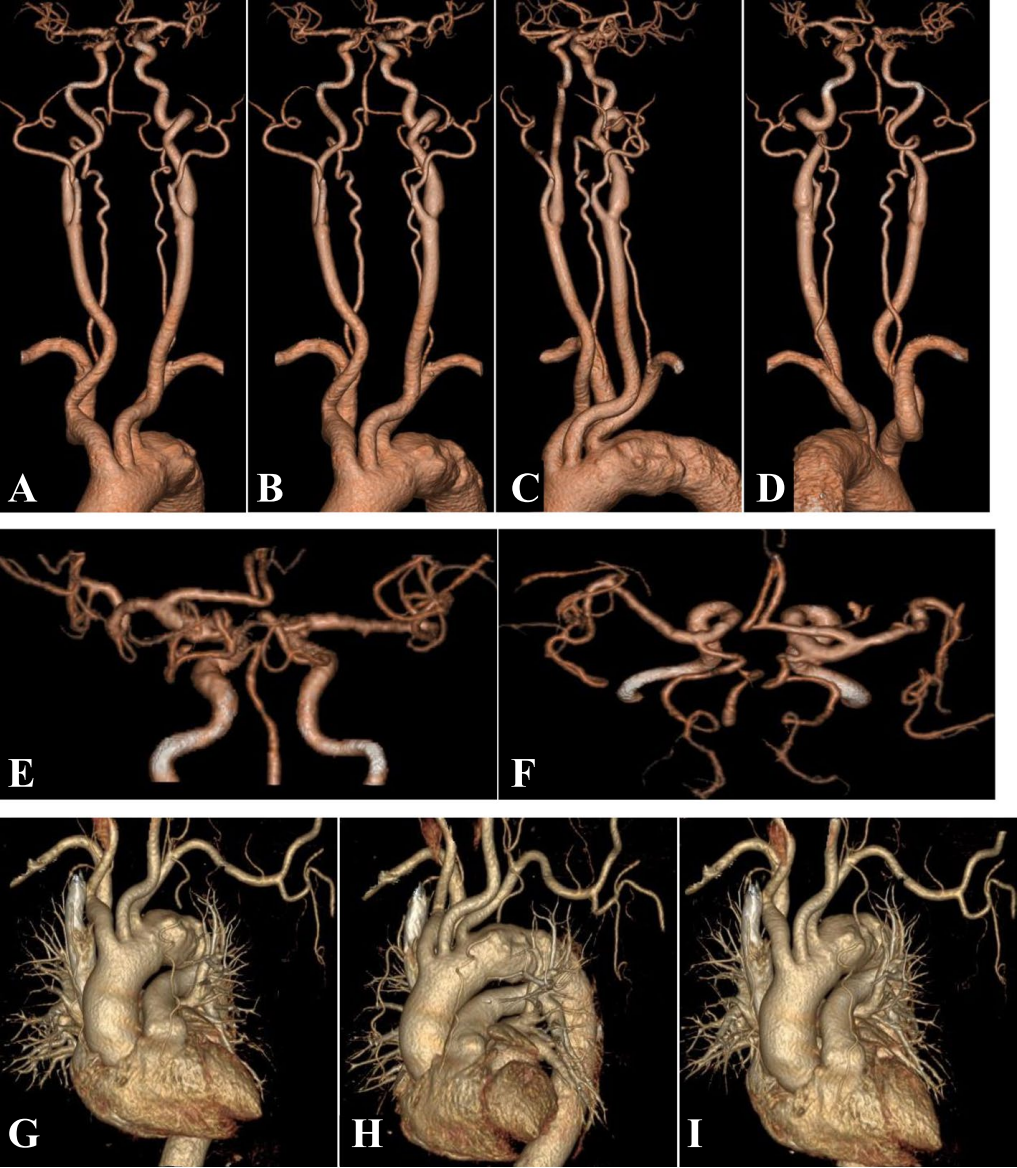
A-I: Head and neck CTA (computed tomography angiography), and chest CTA: noncalcified plaque of the aortic arch with mild stenosis and multiple penetrating ulcers, noncalcified plaque and mild stenosis of the descending aorta; Noncalcified plaque with mild luminal stenosis in the proximal segment of the left common carotid artery and the initial segment of the left internal carotid artery; mixed plaque in the left carotid sinus and mild luminal stenosis; Calcified plaque in the right carotid sinus and the C4–6 segment of the right internal carotid artery; and mild stenosis of the basilar artery
